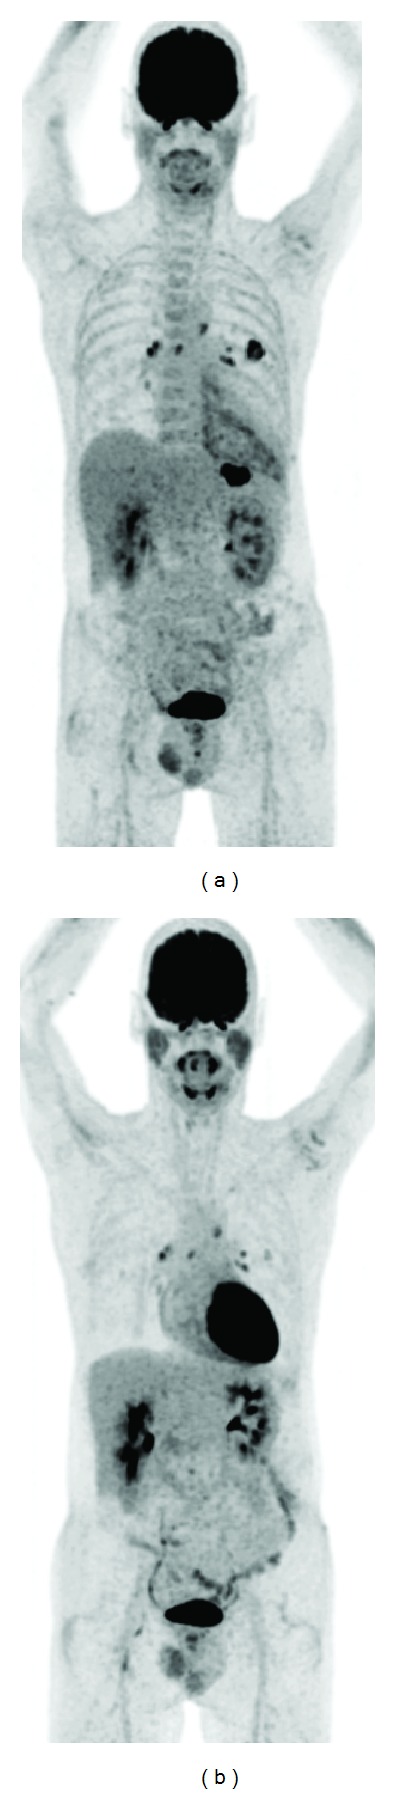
FDG-PET of a neutropenic patient (due to leukemia) with a fungal infection (aspergillosis) before (left image) and during antifungal therapy (right image) resulting in a decrease in FDG uptake in the lung lesions. Because on the last FDG-PET scan (right image) there is still no complete remission, the patient is still treated with antifungal drugs.
